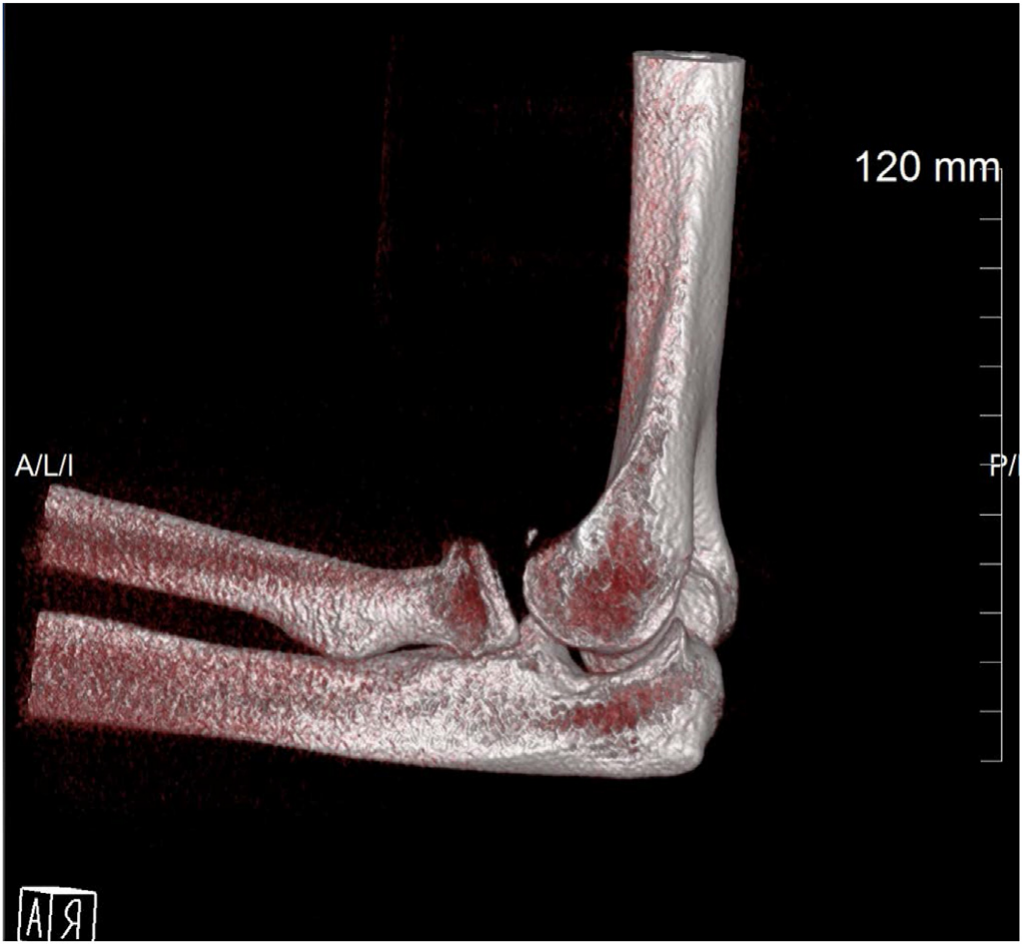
Preop 3D CT image. *3D*, three-dimensional; *CT*, computed tomography.
